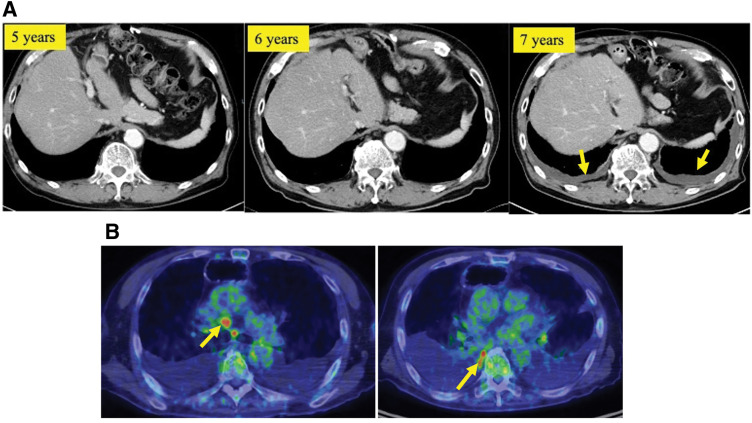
(A) Chest CT scan shows no appearance suspected of recurrence of esophageal squamous cell carcinoma until 6 years postoperatively. However, bilateral pleural effusions appeared on the CT scan conducted during the 7th postoperative year. (B) ^18^F-fluorodeoxyglucose PET/CT was conducted 3 months after the last CT scan and confirmed recurrence in multiple mediastinal lymph nodes and the right lateral pleura. The yellow arrows indicate bilateral pleural effusions on CT (A) and abnormal FDG uptake in mediastinal lymph nodes and the right pleura on PET/CT (B).
